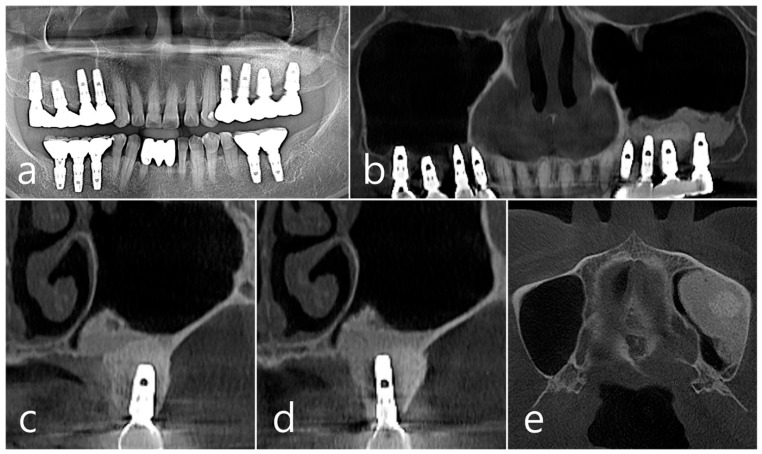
(a,b) In panoramic radiography and panoramic image of CBCT taken 5 years after GBR, previous radiolucent images were no longer observed; (c) Well-consolidated bone graft was observed around the #26 implant; (d) Bone formation and corticalization on the buccal side of the #25 implant was well achieved; (e) A well-consolidated bone graft was observed in the CBCT axial image.
